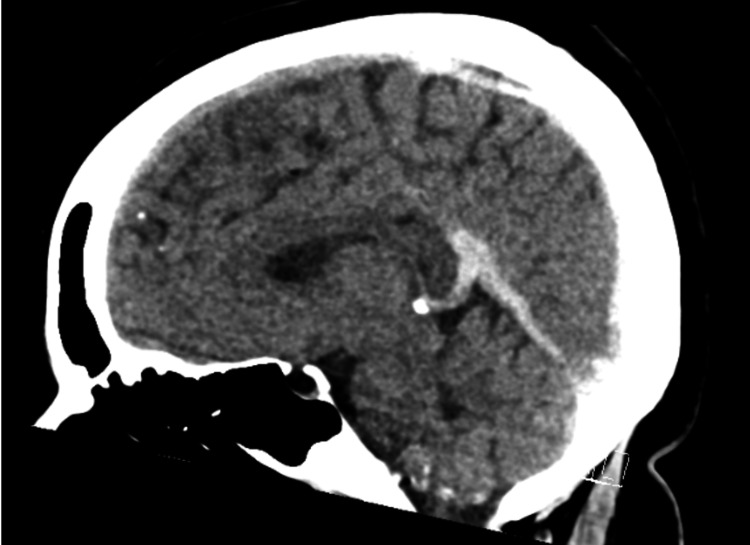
Sagittal View of Head CT Imaging showing hyperdense straight sinus and dorsal aspect of sagittal sinus compatible with thrombus.
